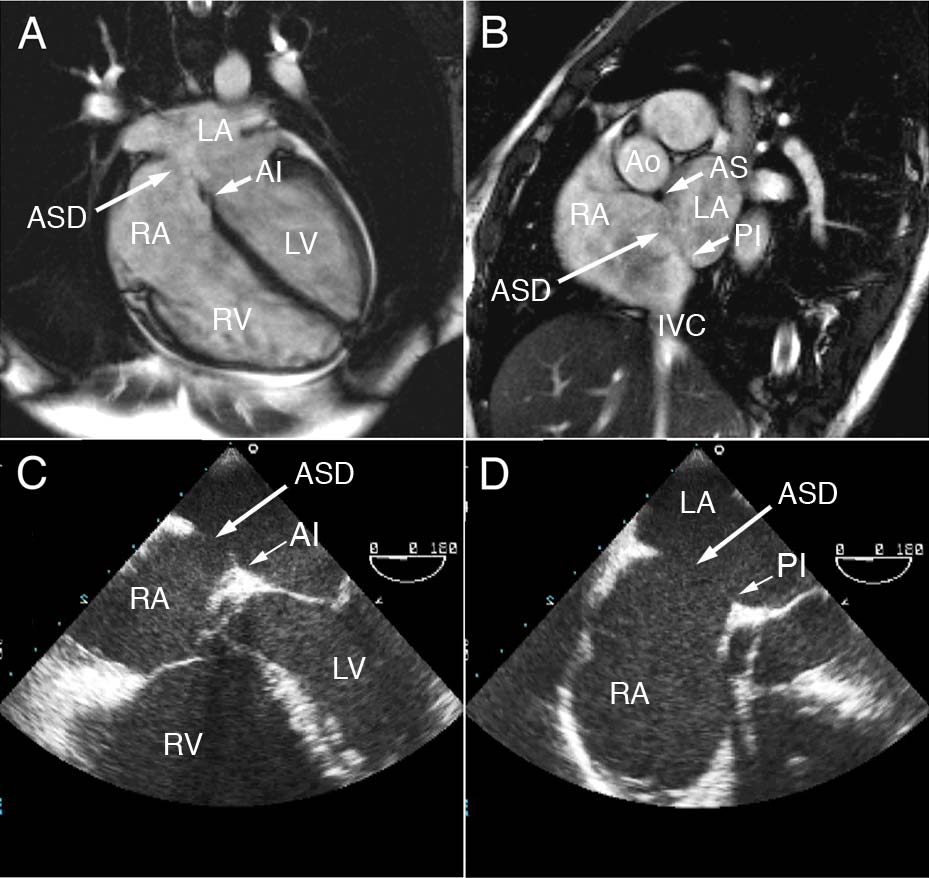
Atrial septal defect (ASD) and margins imaged with CMR (panel A - 4 chamber view, panel B - modified biatrial short axis view) and transoesophageal echocardiography (panel C - 4 chamber view, panel D - biatrial short axis view). Margins are denoted with AS anterior superior rim, AI anterior inferior, PS posterior superior, PI posterior inferior.
